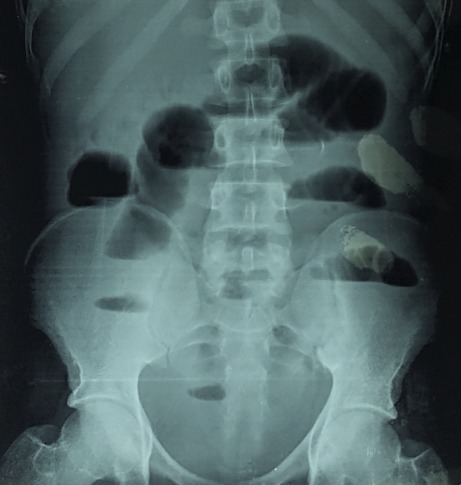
radiographie de l’abdomen sans préparation montrant des niveaux hydo-aériques de type grélique
